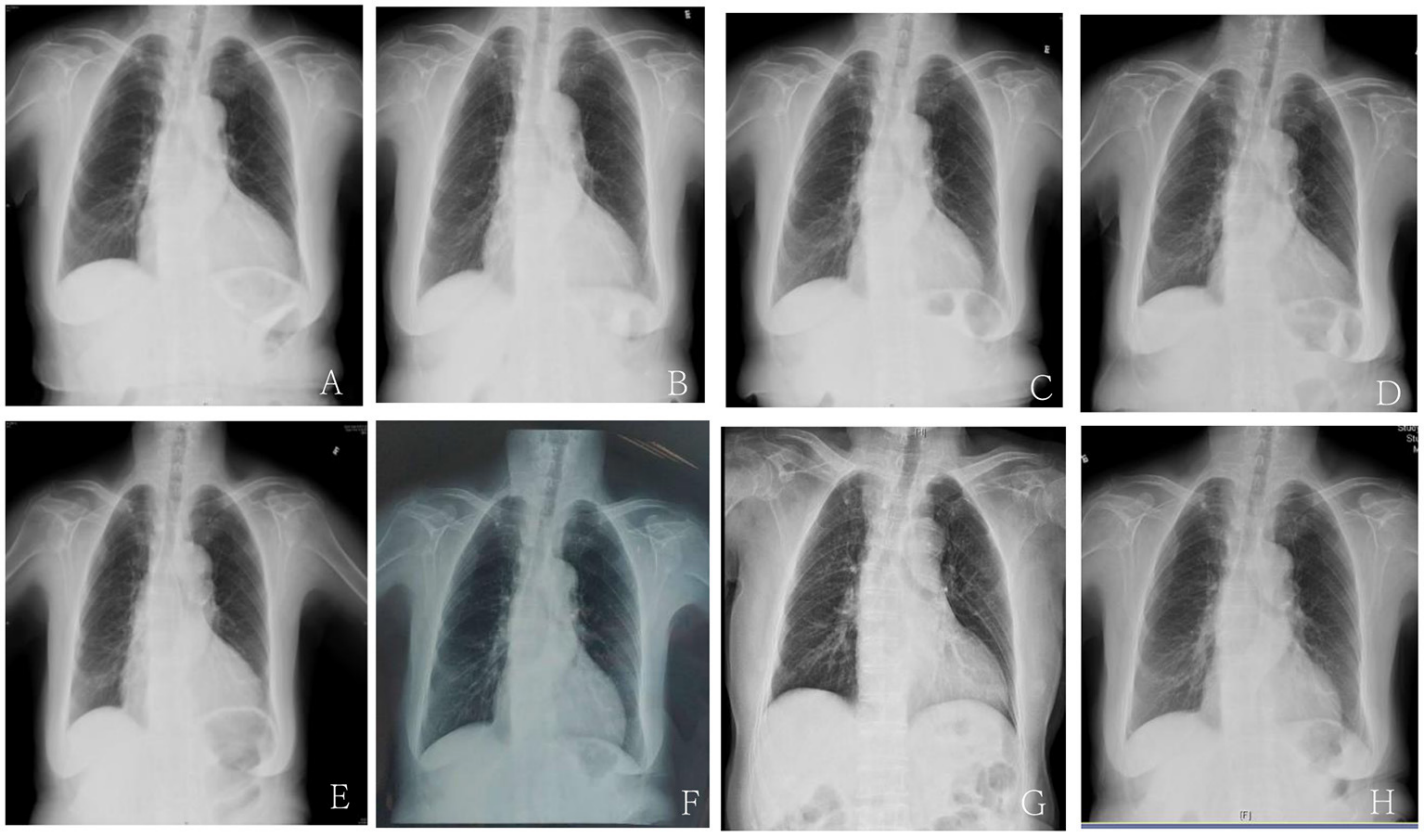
The cardiothoracic ratio (CTR) of chest X-ray before and after treatment with Sacubitril/valsartan. (A) The CTR of chest X-ray upon admission due to the first episode of HF before treatment with sacubitril/valsartan was 0.6. (B) The CTR of chest X-ray at 2 months after sacubitril/valsartan treatment was 0.57. (C) The CTR of chest X-ray at 4 months after sacubitril/valsartan treatment was 0.56. (D) The CTR of chest X-ray upon admission due to the second episode of HF 8 months after dosage reduction of sacubitril/valsartan treatment was 0.64. (E) The CTR of chest X-ray at 11 months after sacubitril/valsartan treatment was 0.6. (F) The CTR of chest X-ray at 17 months after increasing the dosage of sacubitril/valsartan treatment was 0.56. (G) The CTR of chest X-ray at 25 months after sacubitril/valsartan treatment was 0.57. (H) The CTR of chest X-ray at 40 months after sacubitril/valsartan treatment was 0.55.
